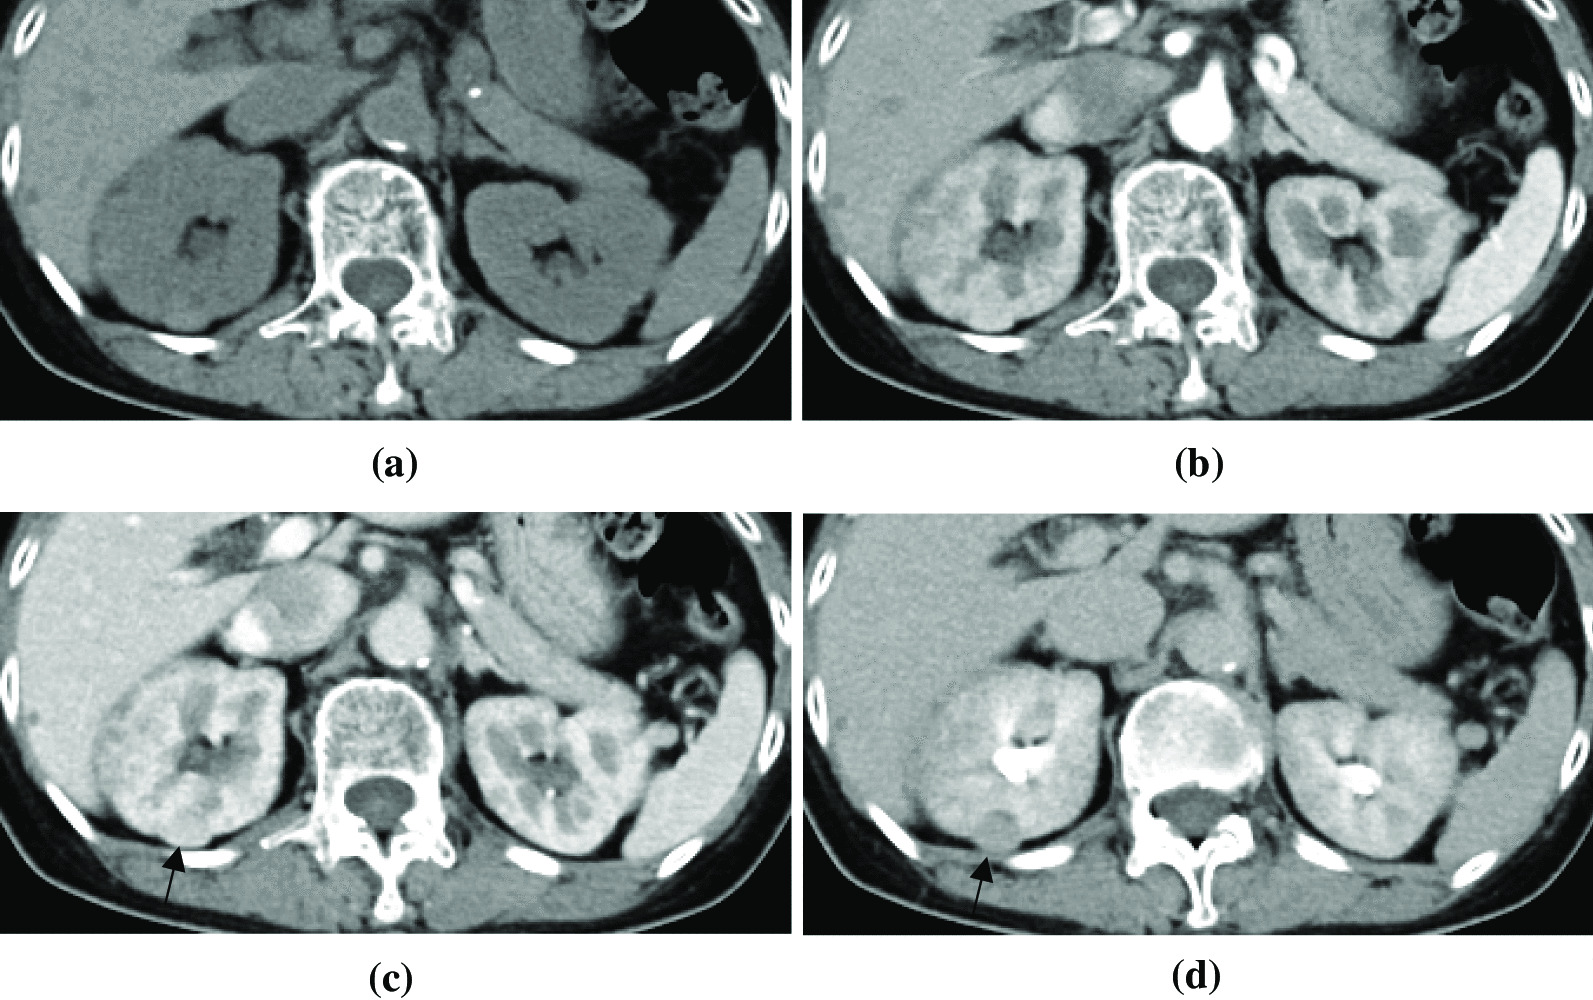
Iodine-enhanced CT images acquired in October 2018. a On unenhanced CT images, a slightly hyperdense and exophytic-growing nodule was observed (pathological analysis showed epithelioid angiomyolipoma) at the posterior aspect of the right kidney. b In arterial-phase CT images, the epithelioid angiomyolipoma was significantly enhanced. In addition, there were multiple small renal nodules (pathological analysis showed papillary adenomas) with poor enhancement in the bilateral kidneys. c, d On nephrographic and excretory-phase computed tomography images, the epithelioid angiomyolipoma (black arrow) was hypodense relative to the adjacent renal cortex. The papillary adenomas were iso- to hypodense in the renal cortex in the excretory phase
